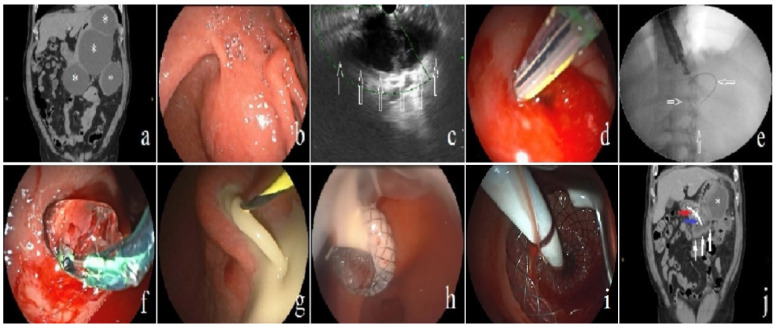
Placement of lumen-apposing metallic stent (LAMS) in the walled-off necrosis (WON) cavity. (a) CT demonstrating multi-lobulated WON cavities (asterisk). (b) Endoscopy demonstrating bulging of the gastric lumen. (c) Determining the WON cavity and selecting an appropriate drainage site with echoendoscope in a patient exhibiting no signs of bulging on the intestinal lumen (arrow). (d) Cavity puncture was performed with a needle knife sphincterotome. (e) Subsequently, a guidewire was carefully introduced into the cavity to facilitate further procedures (arrow). (f) Balloon dilatation was performed on the cystogastrostomy tract. (g) Purulent fluid was seen flowing into the lumen. (h) LAMS was placed to extend into the WON cavity. (i) To prevent migration, a double pigtail stent was positioned through the LAMS, extending into the cavity. (j) Three days post-procedure, the CT demonstrated the LAMS (red arrow), double pigtail stent (blue arrow), a visibly reduced volume of the drained WON cavity (white arrow), and another WON cavity (asterixis) that remained unaffected by endoscopic drainage.
